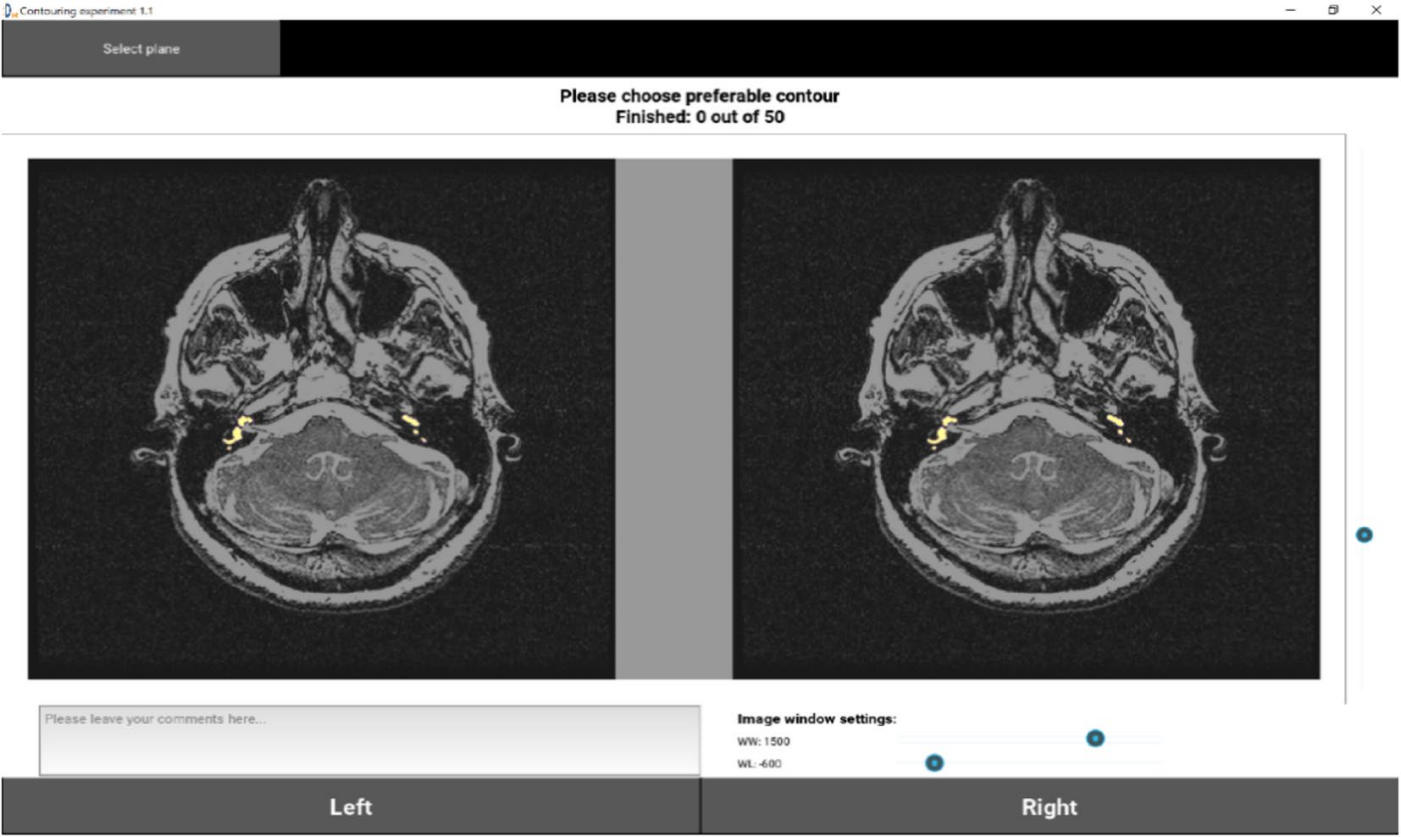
Example automated and manual segmentation overlaid on MRI volume as displayed by the software.
